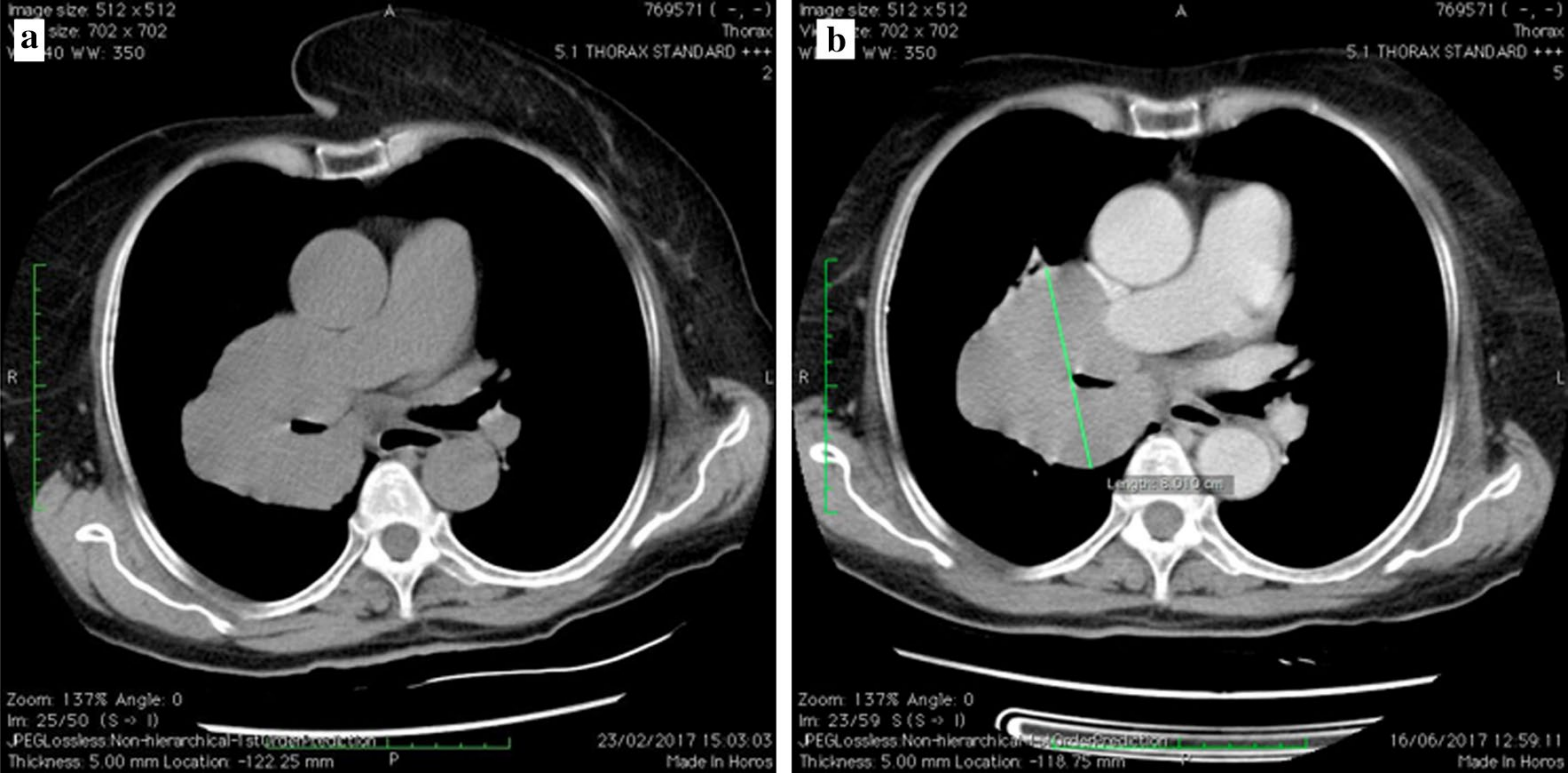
CT-scan after 1 month and half of treatment showed a well demarcated and homogeneous right proximal tumor with a bronchial compression. The tumor measures about 8 cm (a). After 5 months of treatment, the tumor presents the same features (b)
